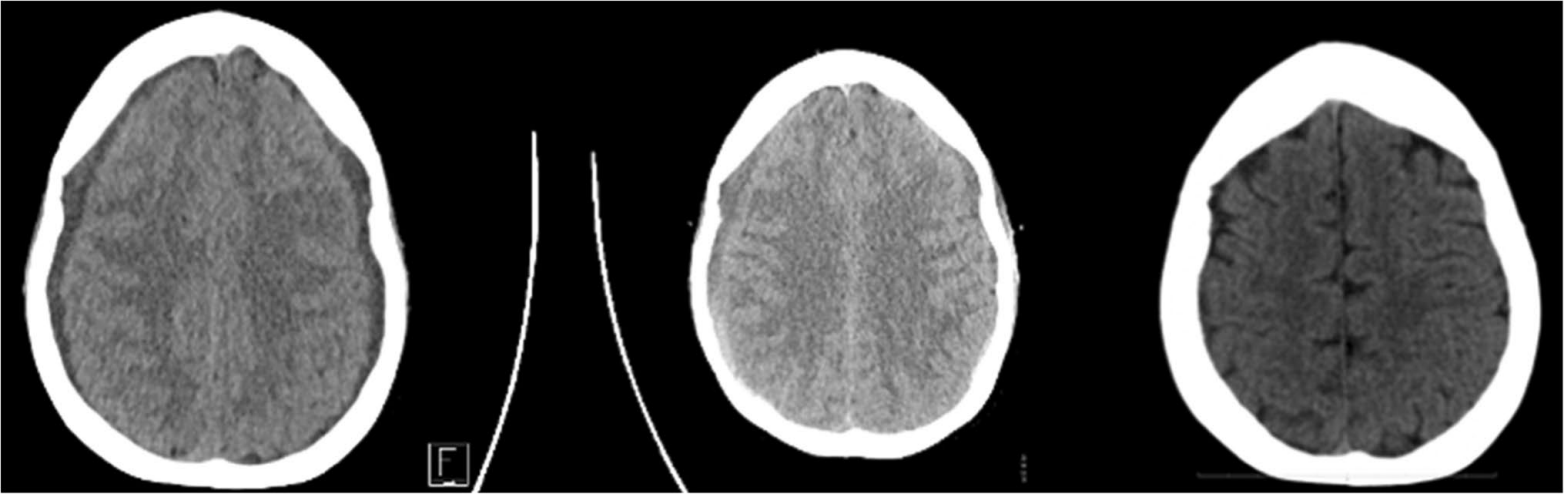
Postoperative CT scan at one, 2 and 3 months (from left to right) showing the complete resolution of the subdural hematomas
